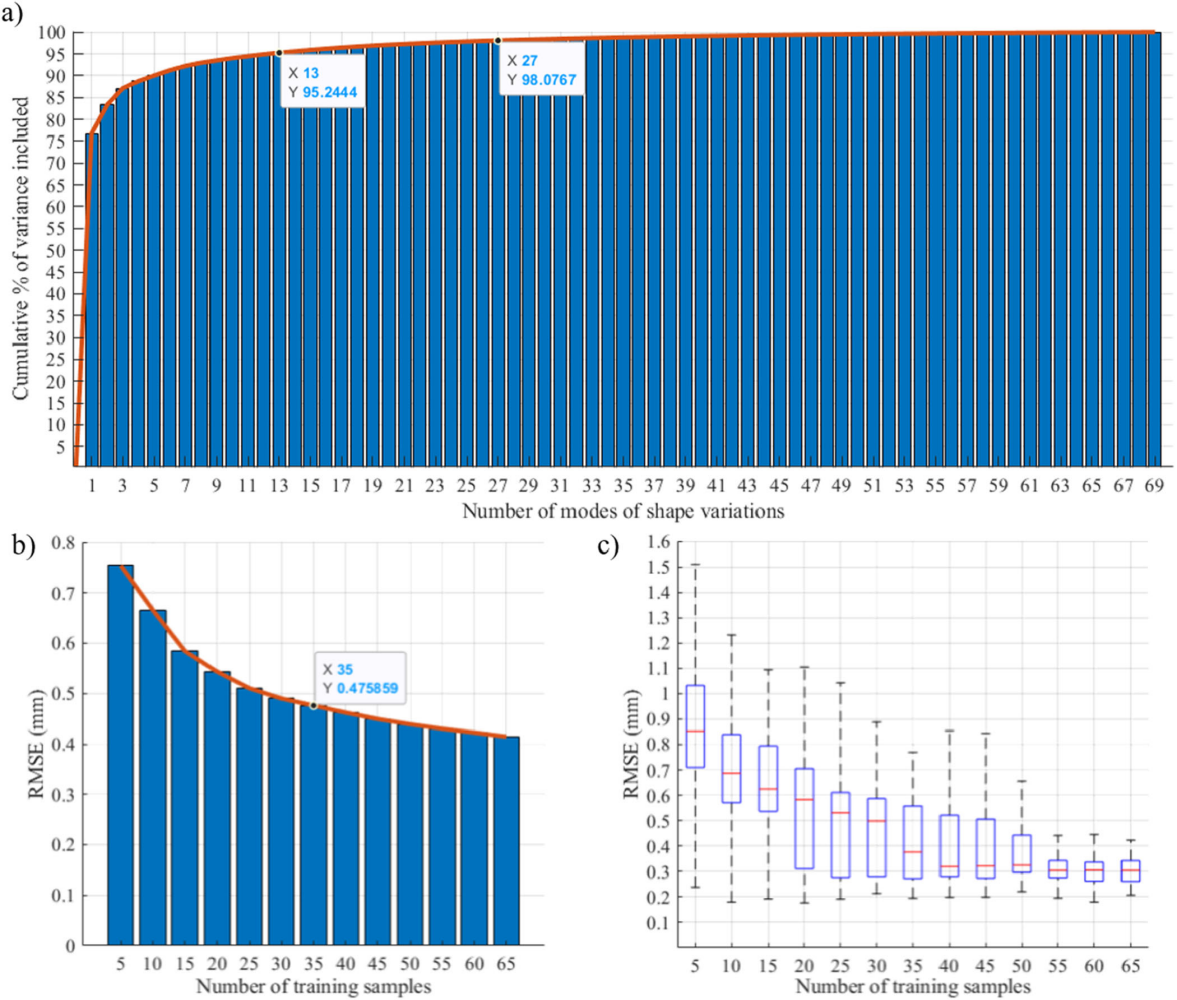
Results of the Statistical Shape Model (SSM) compactness, accuracy, and generalisation ability evaluation. (a) SSM compactness is presented as the cumulative variation of data included in the model based on the number of shape modes. The first 13 and 27 modes of shape variation account for 95% and 98% of the cartilage shape variation within the studied population, respectively. (b) Accuracy of the SSM based on the number of training samples; using the first 35 modes of variation, the accuracy of the SSM reached a value (0.48 mm) below the resolution of the magnetic resonance imaging (MRI) images (0.5 mm) for the first time, and (c) generalisation of the SSM based on the number of training samples. The generalisation ability of the SSM reached a value (0.30 mm) below the resolution of the MRI images (0.5 mm) for the first time when the first 55 modes of shape variation were used.
